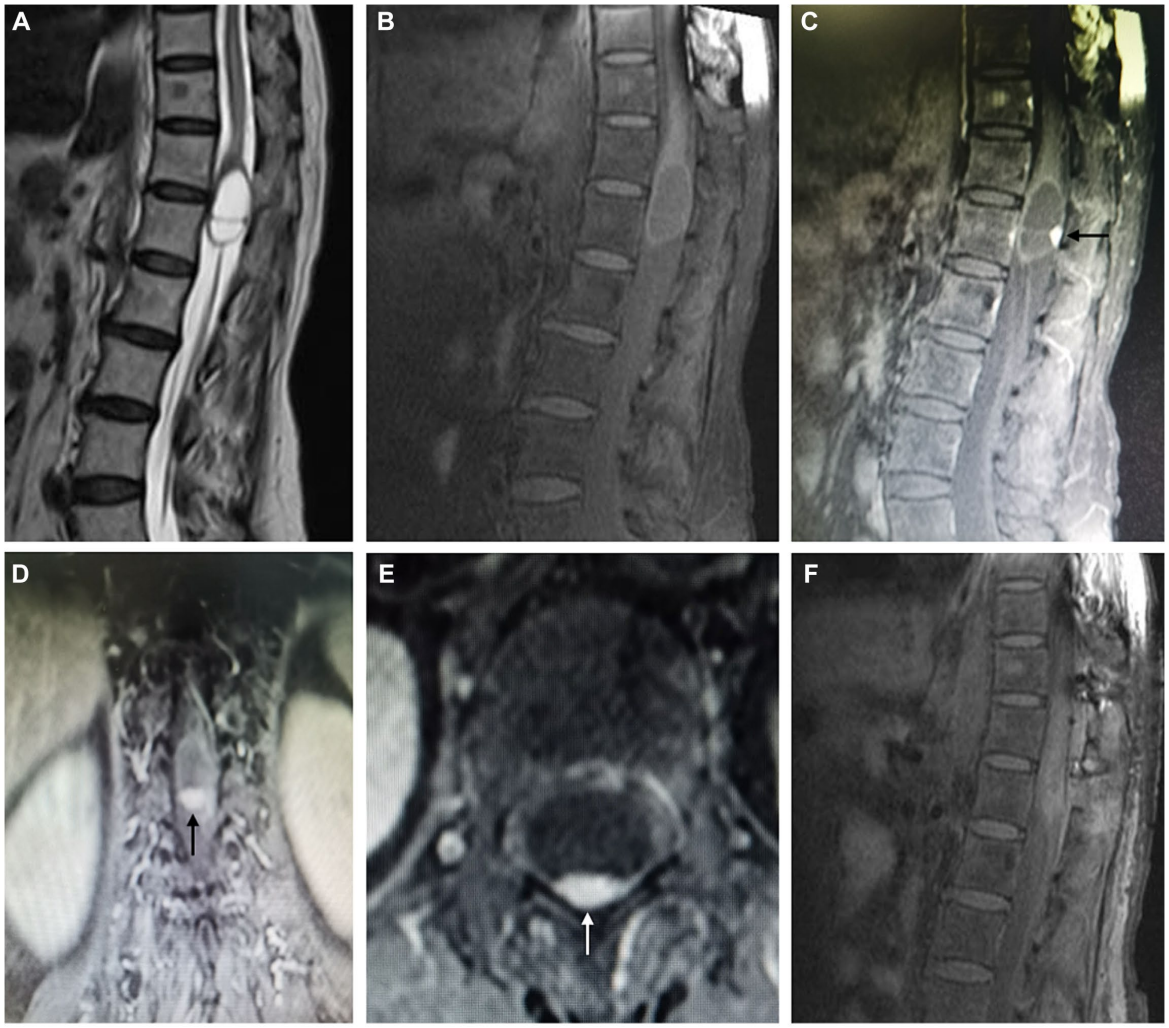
Magnetic resonance imaging. Preoperative MR T2 and T1-weighted images demonstrate an intradural septate cystic mass with a similar intensity of cerebrospinal fluid located at the terminal end of the conus medullaris, with a small posterior nodular lesion (A,B). Preoperative MR T1-weighted contrast-enhanced images demonstrate homogenous enhancement of posterior nodule (arrow) and cystic wall (C–E). Postoperative MR T1-weighted contrast-enhanced image demonstrates the gross total resection of the mass (F).
